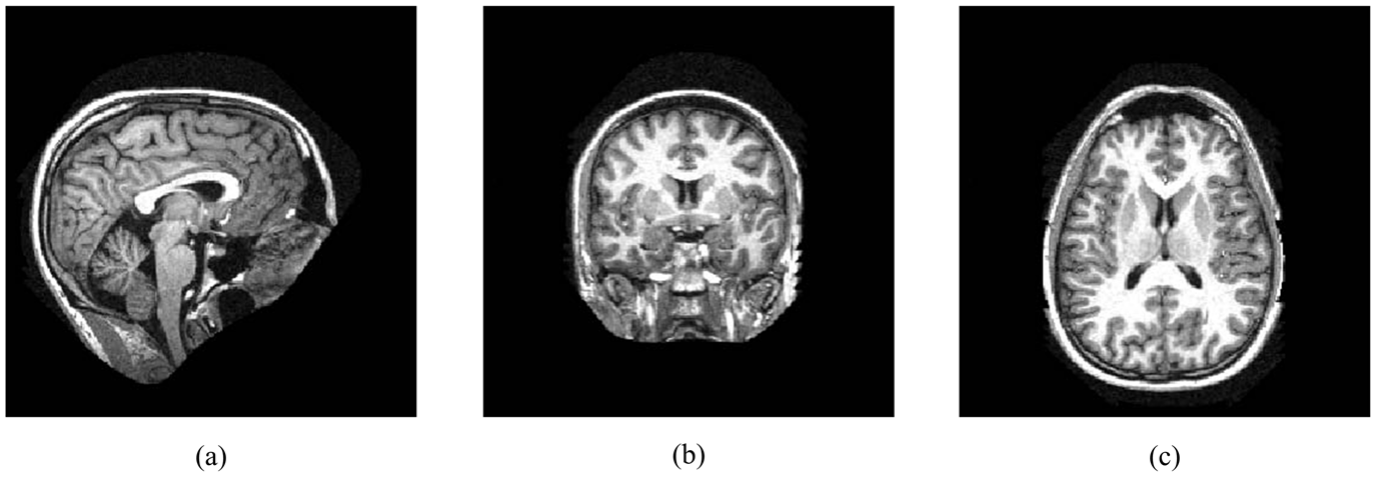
An example of an MR image in the OASIS database. (a) A sagittal section, with the front (anterior) of the head at the right and the top of the head shown at the top. This orientation shows an image as if the subject were being viewed from the right. (b) A coronal section, with the top (superior) of the head displayed at the top and the left shown on the left. This orientation shows an image as if the subject were being viewed from behind. (c) An axial section, with the front (anterior) of the head at the top and the left shown on the left. This orientation appears as if the subject were being viewed from above.
